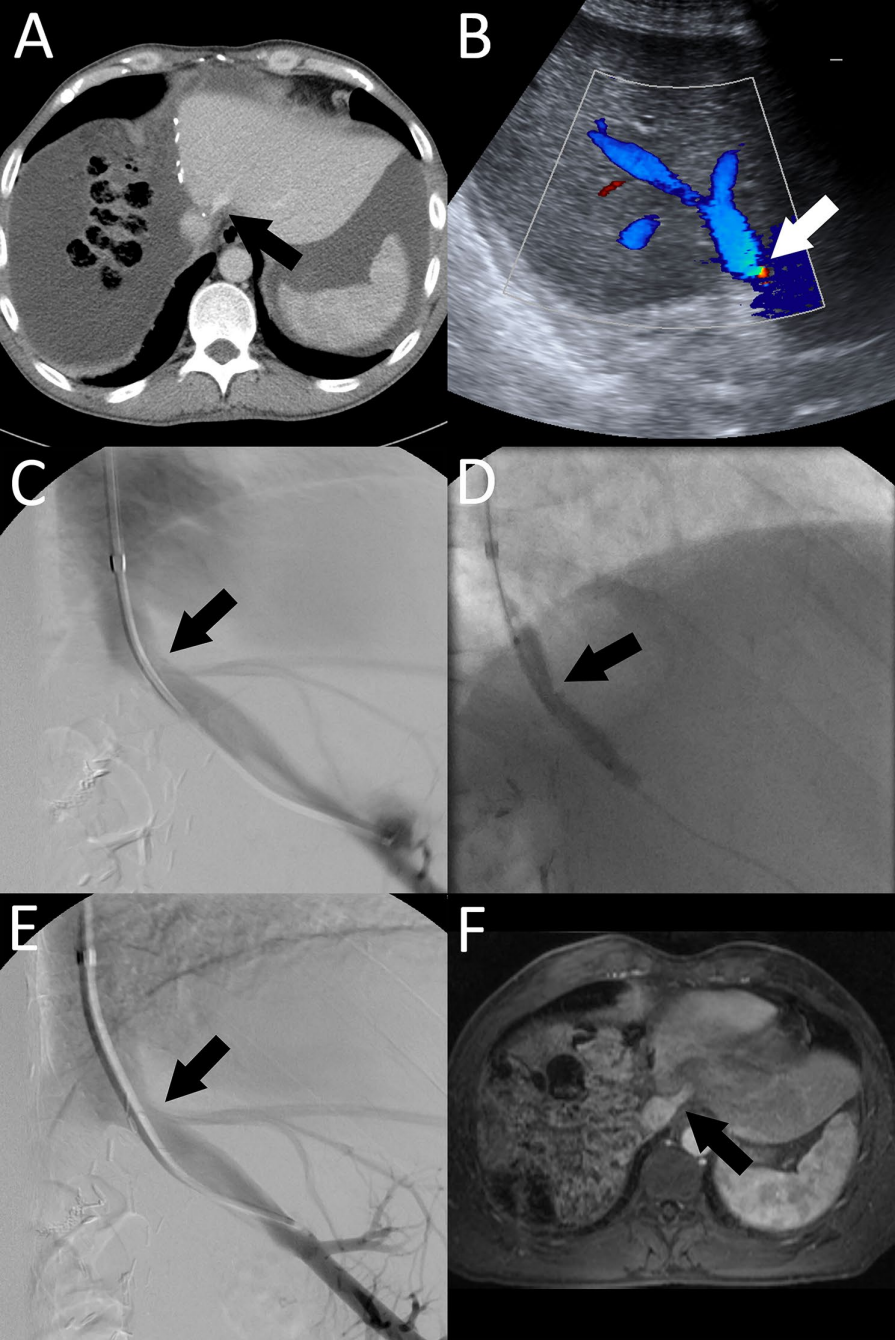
46-year-old male (patient no. 2) presenting with hepatic venous stenosis after extended right hepatectomy for cholangiocarcinoma. A: Axial postsurgical CT scan suggesting severe hepatic venous stenosis (arrow). B: Ultrasound exam confirms significant flow acceleration in the area of the suspected hepatic vein stenosis (arrow). C: Stenosis was found during venography and treated by balloon angioplasty using a 10 mm compliant balloon (D). E: Post-interventional result after balloon angioplasty without evidence of residual stenosis. F: MRI scan four months post intervention demonstrates a patent hepatic vein
